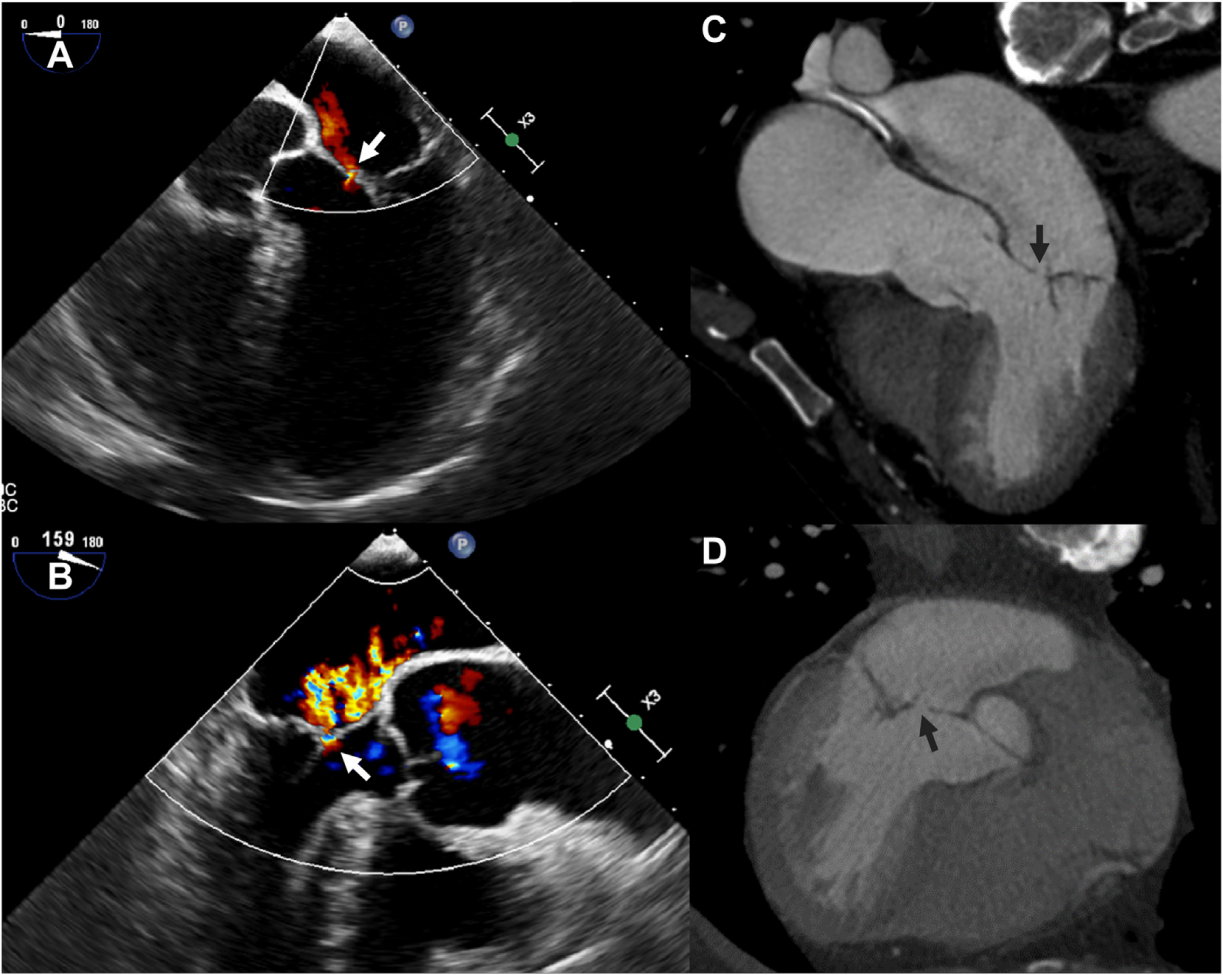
Perforation of the anterior mitral valve leaflet in a patient with native mitral valve endocarditis. The transesophageal echocardiography (A and B) images show the Doppler jet traversing the anterior mitral valve leaflet (arrow) indicating a perforation. The computed tomography images (C and D) show a focal discontinuity (arrow) in the valve leaflet compatible with a perforation.
